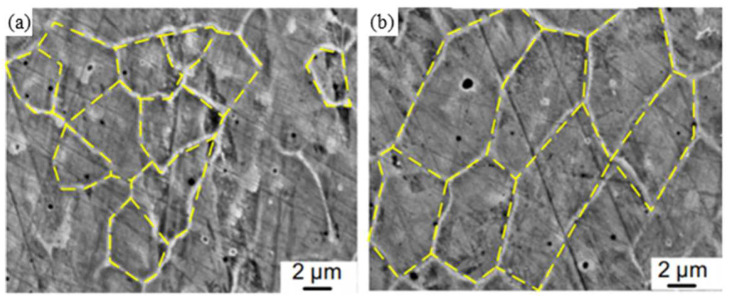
Effects of ultrasonic vibrations on the grain size of AISI 630 thin walls fabricated by LENS: (a) with ultrasonic vibrations; (b) without ultrasonic vibrations [68].
